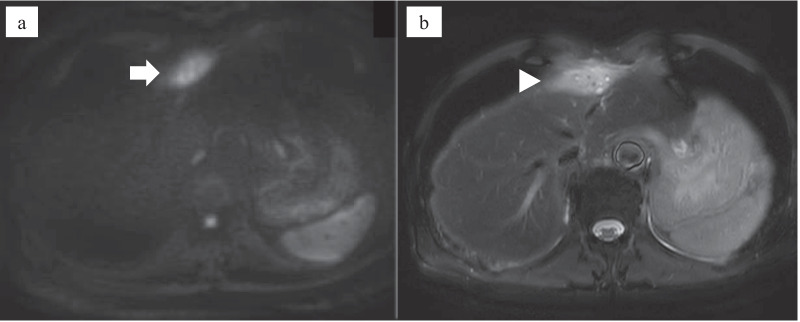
Magnetic resonance imaging (MRI) of the falciform ligament abscess at the time of the first admission. a The falciform ligament mass showed high signal intensity on diffusion-weighted images (white arrow). b Small high-intensity areas within the mass were visible on T2-weighted images (white arrowhead)
